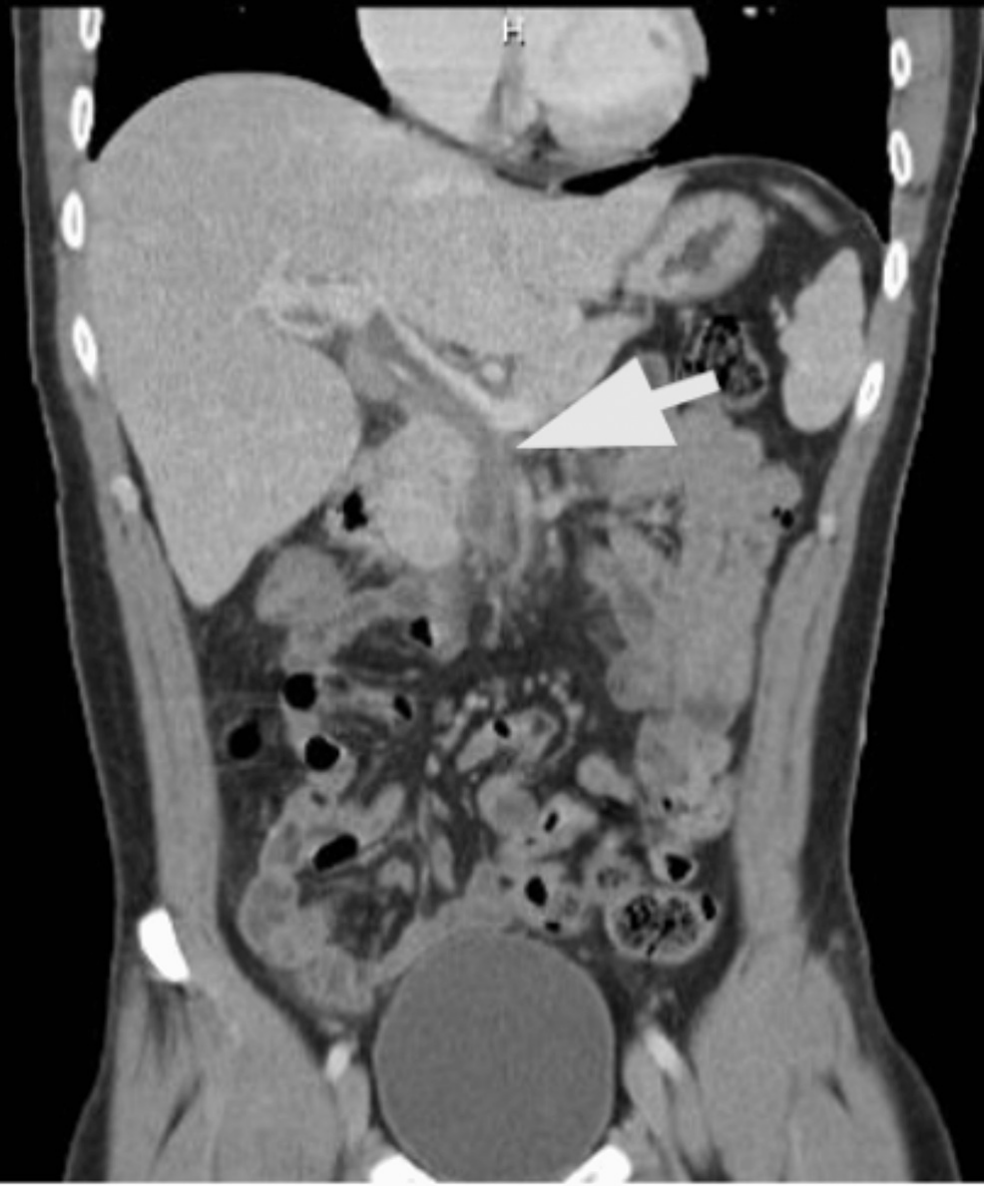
Contrast enhanced CT of the abdomen and pelvis showing venous thrombosis within the superior mesenteric vein and portal vein
